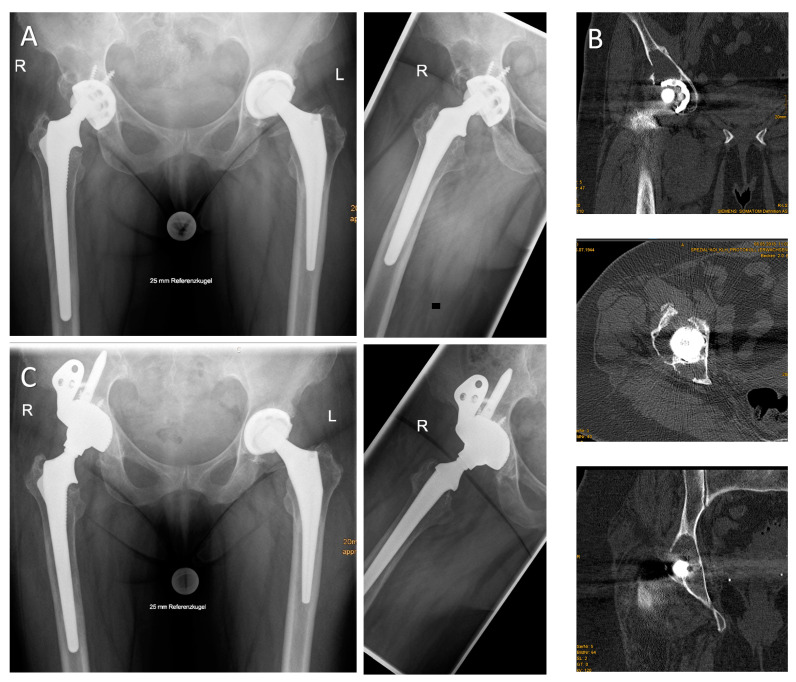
(A) A.p. and axial radiographs of an 81-year-old female patient with large periacetabular osteolyses due to wear debris 17 years after primary hip arthroplasty. (B) Multiaxial CT analyses revealed insufficient stability of both the anterior and posterior column. Intraoperatively, the large iliac defect was contained by an egg shell-like outer cortex. More than 60% of the acetabular rim was deficient. The iliac defect was filled with impacted allogenic bone chips and the asymmetric cup was augmented with a 50-mm intramedullary iliac press-fit stem and multiple screws. (C) Radiographic imaging 24 months postoperatively revealed a stable implant with well-integrated bone grafts (HHS: 46 preoperatively, 66 postoperatively; OT 150 min).
